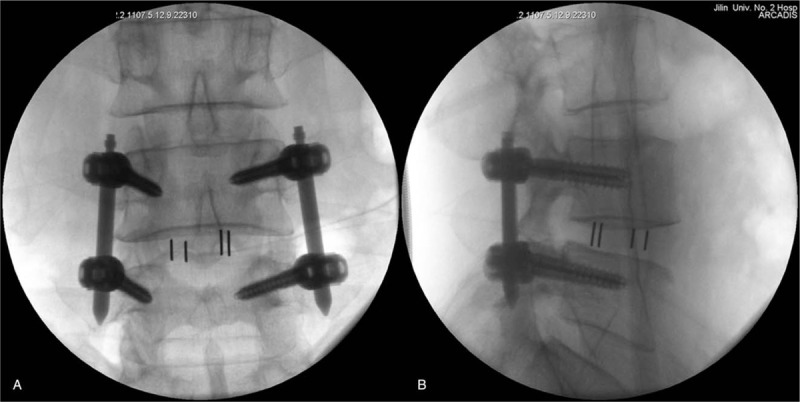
Anteroposterior (A) and lateral (B) radiographs were taken postoperatively and showed that the Zina percutaneous pedicle screws and the cage were in a good position.
